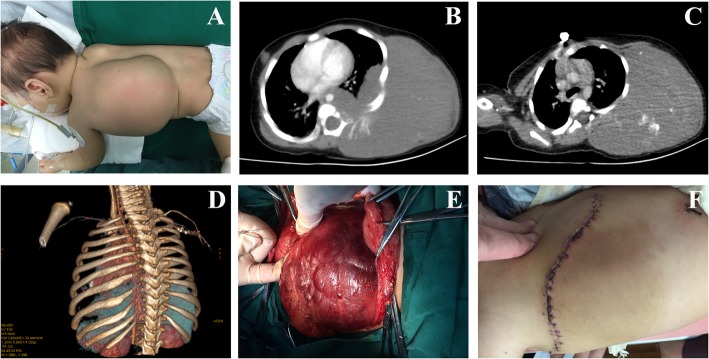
a: A huge mass can be seen on the left side of the back, about 23 cm × 15 cm in size. The mass is cystic and has a sense of undulation, but there is no pulsation. The mass is located under the skin and the skin color has no obvious change. b: CT scan showed a huge lesion from the chest wall invaded into the left thoracic cavity and thoracic vertebra through the intercostal space. Contrast-enhanced CT scan showed no obvious enhancement within the lesion. C: CT scan showed multiple phleboliths formation in the lesion. D: The three-dimensional reconstruction of CTA only found a small amount of blood-supplying vessels on the left side of the spine, and the whole lesion morphology was invisible. E: In the operation, the mass was identified as VMs with abundant blood supply and thin wall. When surgically separated, the mass was prone to rupture and massive bleeding. F: After the operation, the blood supplying of the flap was good, and the wound healed well
